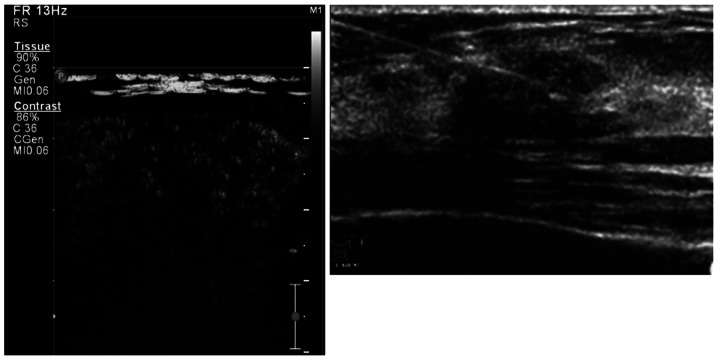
Uneven enhancement by CEUS (pathologically diagnosed as PTC). CEUS, contrast-enhanced ultrasonography; PTC, papillary thyroid carcinoma.
